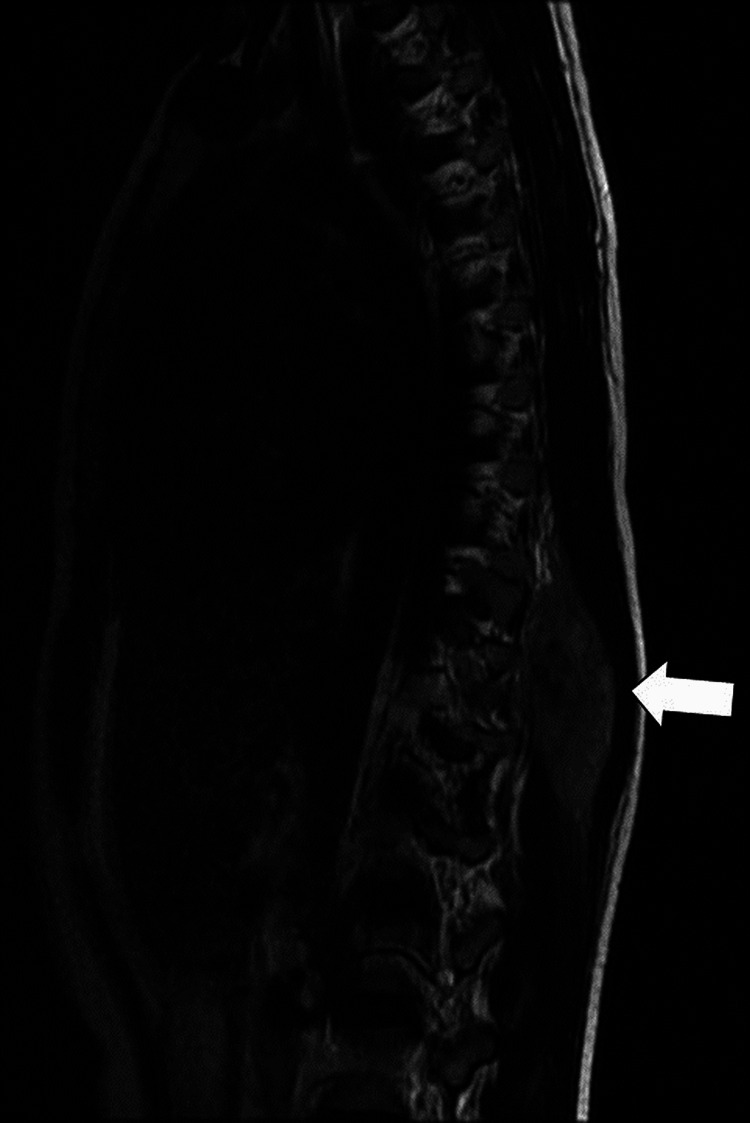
Saggital T2-weighted MRI shows a lesion (arrow) that has heterogeneously high signal intensity.
